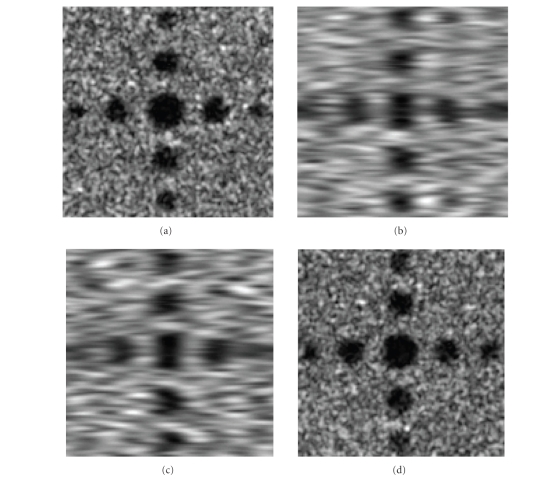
Two reconstructed 3D ultrasound image sets: (a) J
